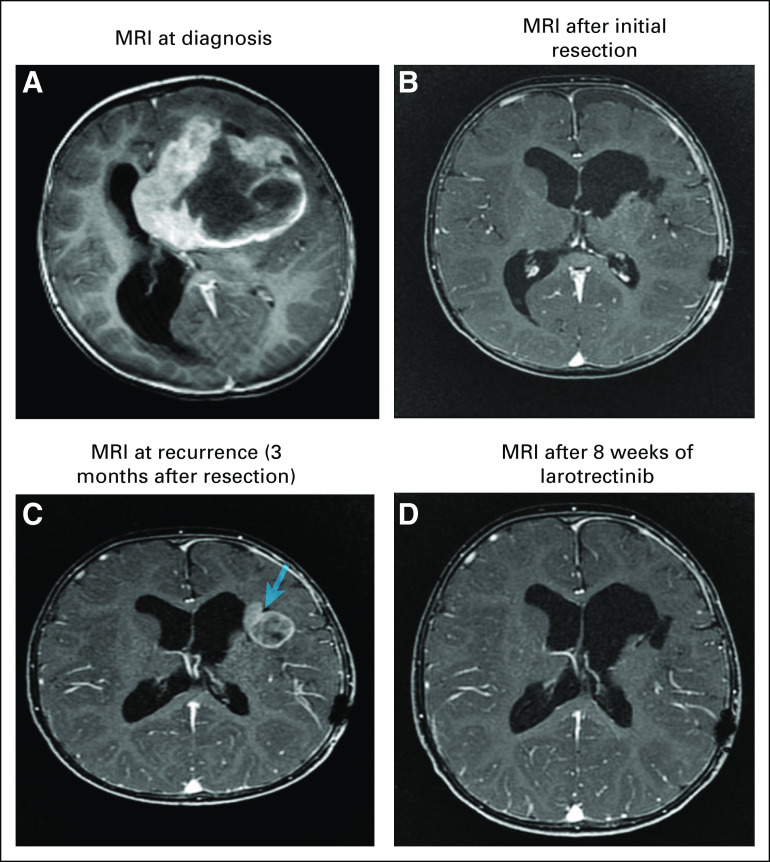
(A) At diagnosis, postgadolinium axial T1-weighted magnetic resonance imaging (MRI) demonstrated a heterogeneous complex mass in the left frontal lobe. (B) After total surgical resection, postgadolinium axial T1-weighted MRI demonstrated total resection of the left frontal mass. (C) At recurrence, postgadolinium axial T1-weighted MRI demonstrated newly appearing mass within the operative bed (arrow) showing intermediate diffusion signal with corresponding contrast enhancement indicating recurrent tumor. (D) Eight weeks after larotrectinib, follow-up postgadolinium axial T1-weighted MRI demonstrated disappearing left frontal mass indicating significant treatment response
